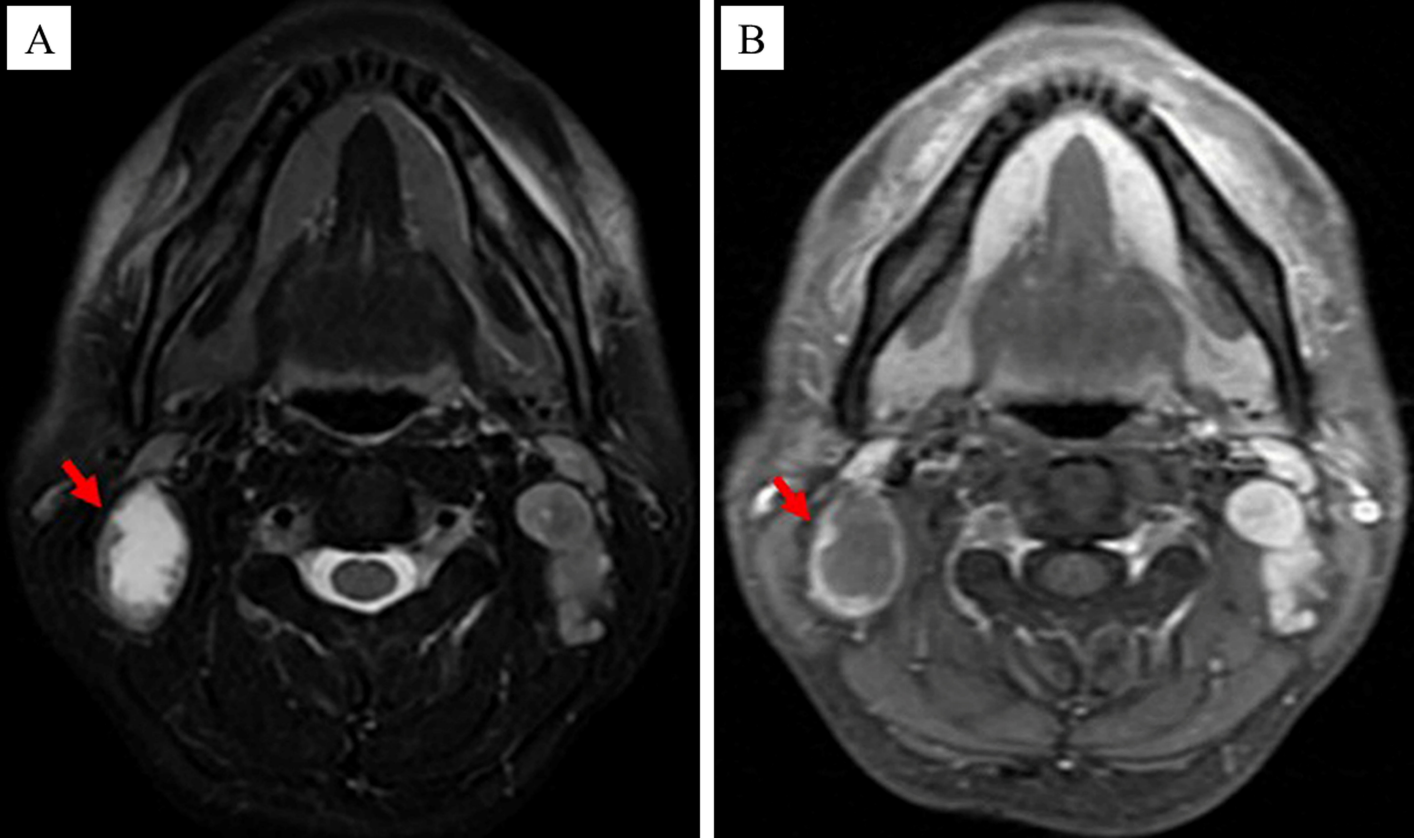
MRI images of the CNN (as indicated by the red arrow). (A) The central region of the CNN showed high signal on the T2-weighted sequence. (B) The central region of the CNN showed low signal on the T1 enhancement sequence.
